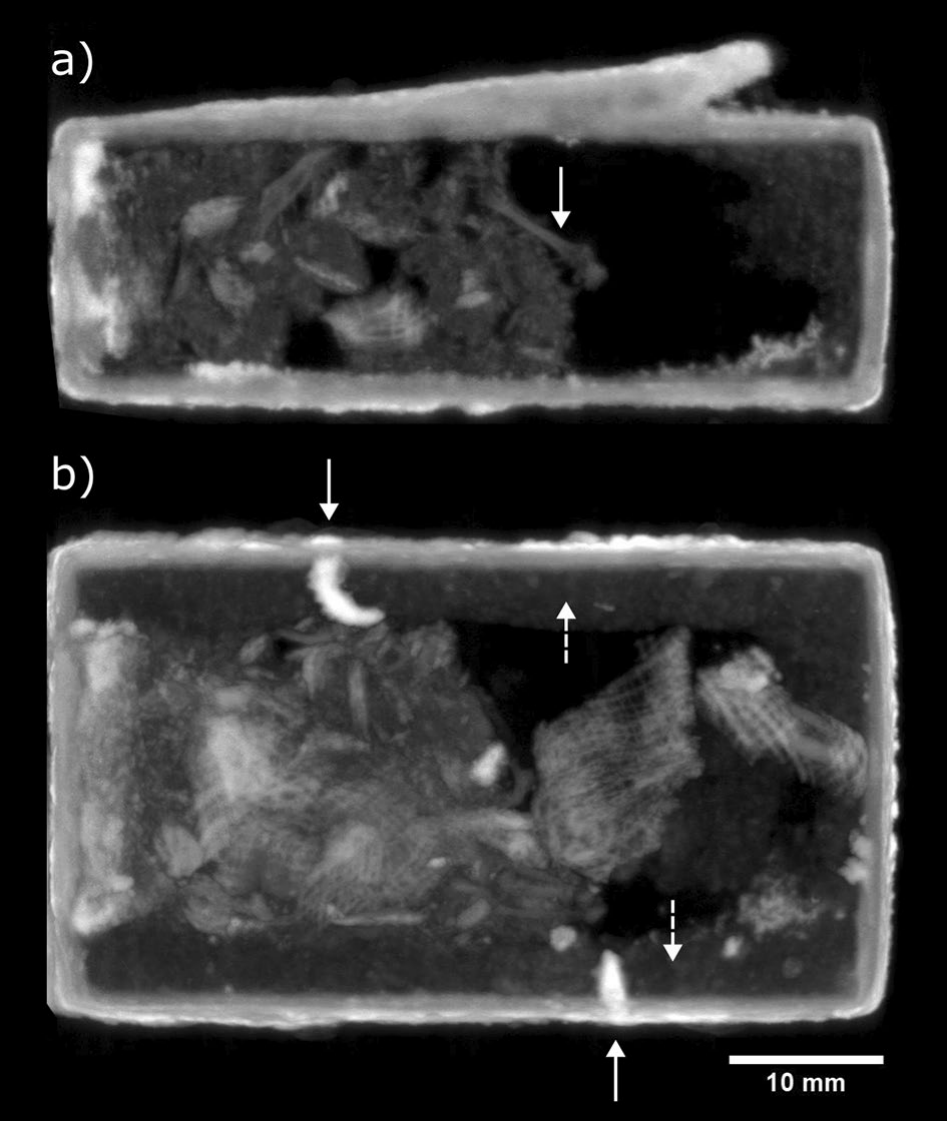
EA27584 multiplanar maximum intensity projection (MIP) neutron CT images: (a) side-view, 1.16 mm thick slice centred 6.1 mm from right side of box, highlighting the presence of an 8.1 mm long bone (solid arrow); (b) top-view, 12.43 mm thick slice centred 8.3 mm from base of box, showing the presence of textile, corroded chaplets (solid arrows), and core material (dashed arrows).
